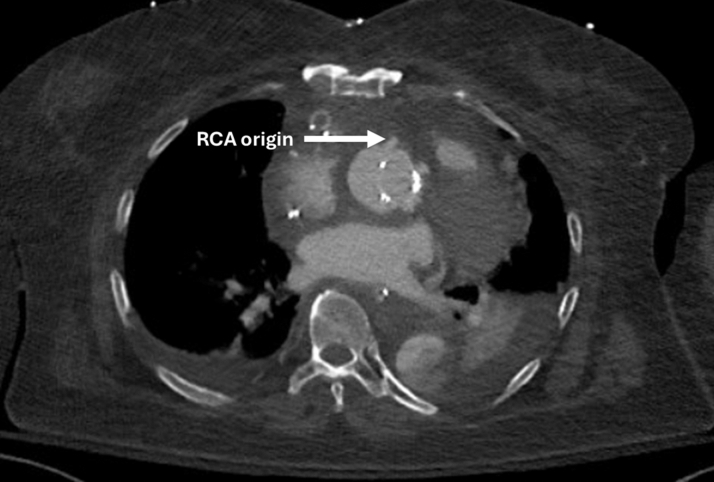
Postoperative CT Scan 1 Week After Surgery Demonstrating a Satisfactory Repair With No Concerning Features The origin of the reimplanted right coronary artery (RCA) is demonstrated.
